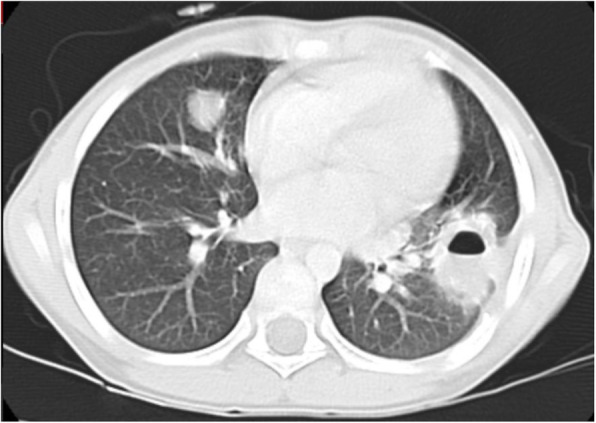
computed tomography of pulmonary hydatid cyst in in base of left lower lobe
